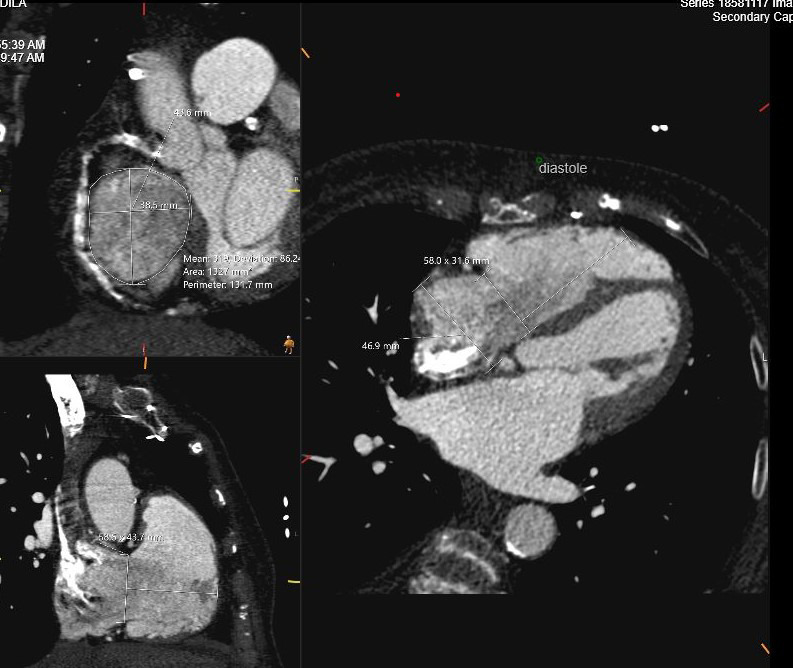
Cardiac CT assessment of TR. Using tricuspid valve protocol
